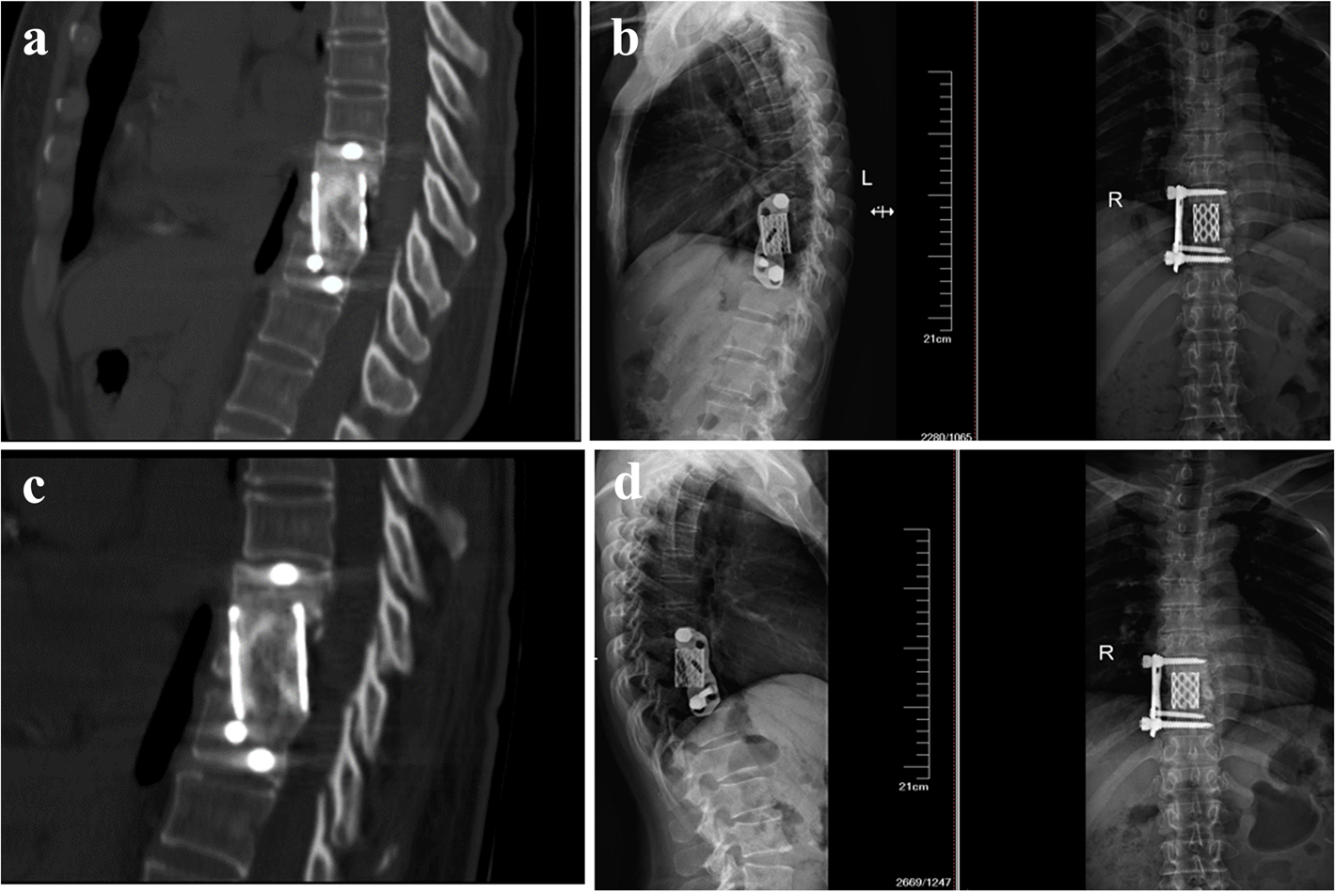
Postoperative DR and CT images showed good position of internal fixation and good bone fusion, respectively. a CT images of postoperative 18 months. b DR images of postoperative 18 months. c CT images of postoperative 24 months. d DR images of postoperative 24 months
